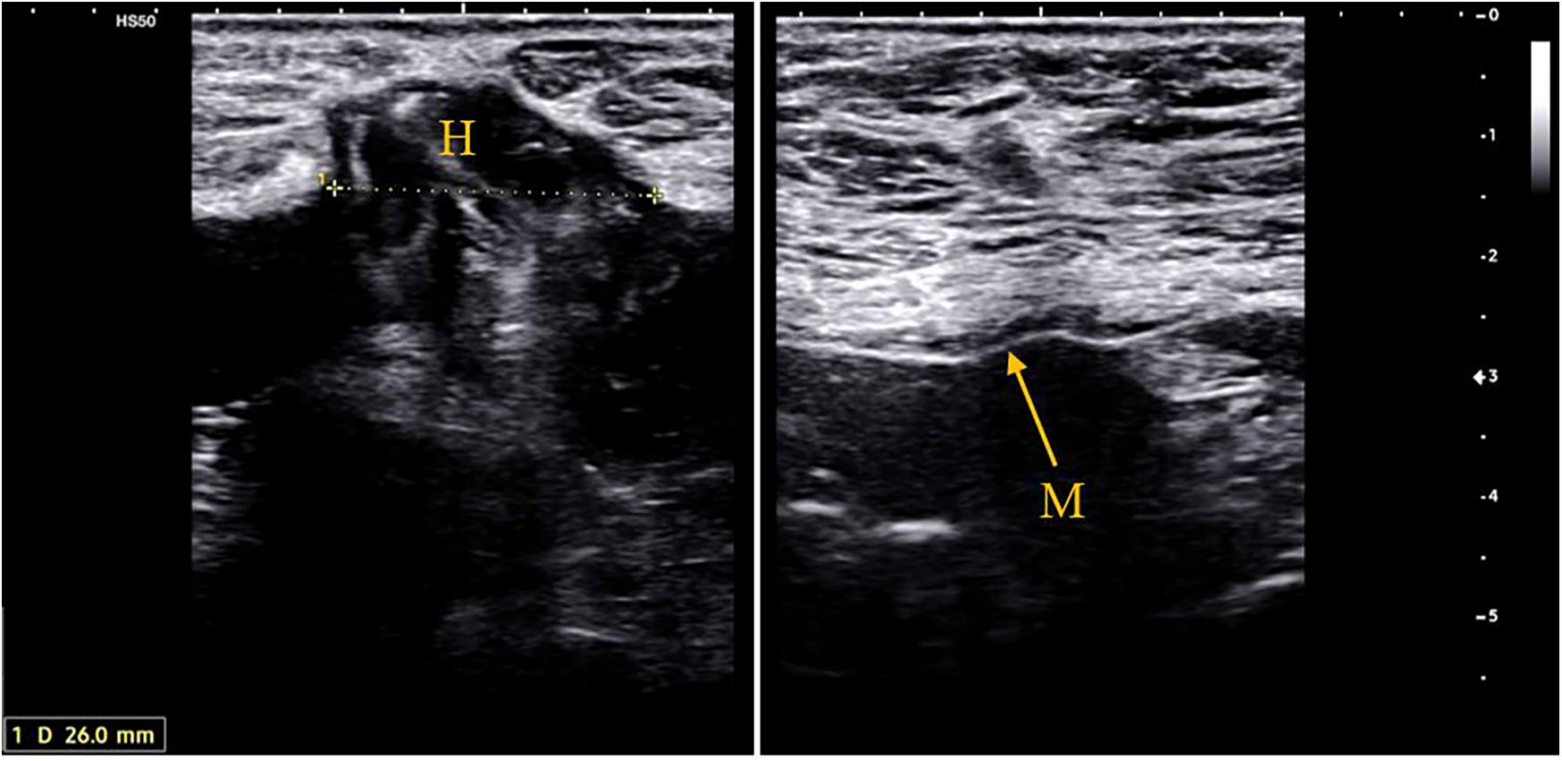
Ultrasound images at 43 months follow-up of a 70-year-old patient who underwent re-laparotomy 18 months after AAA repair for an adenocarcinoma of the gastroesophageal junction. (A) 26 mm. supra-umbilical hernia. (B) Ultrasound image showing the retro-muscular mesh in place M, mesh; H, hernia.
